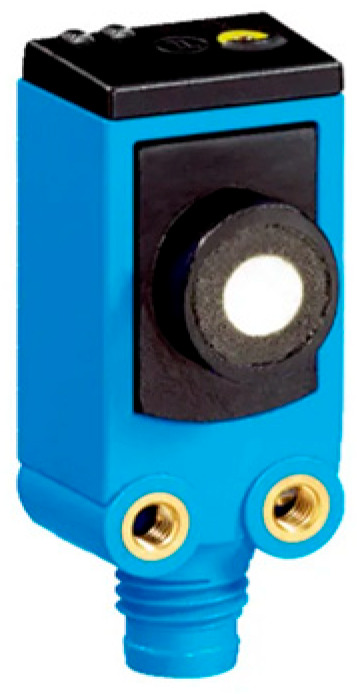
Ultrasonic distance sensor Sick UC4-1334B (SICK AG, Düsseldorf, Germany).
